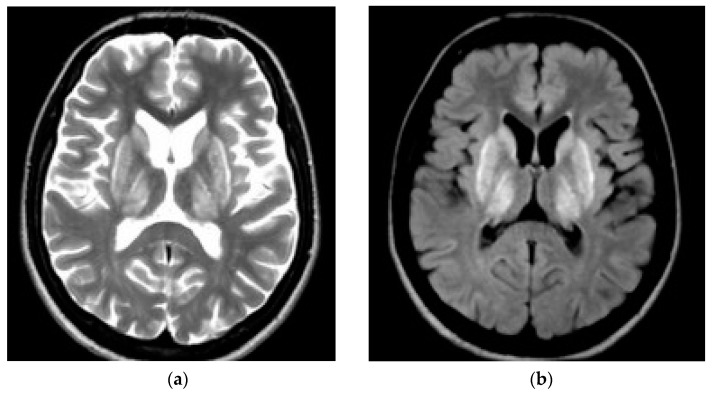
Symmetrical changes visualized in putamen, caudate, and thalamus with bright claustrum sign in brain MRI in T2-weighted sequences (a) and FLAIR sequences (b) (source: own material from 2nd Department of Neurology, Institute Psychiatry and Neurology, Warsaw, Poland).
